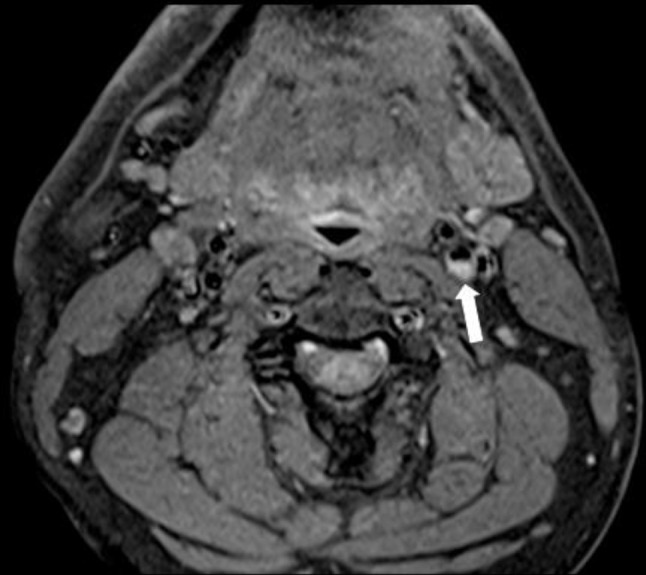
39-year-old male patient who presented with an acute left M1 occlusion with a background history of a prior left MCA infarct. Axial fat-saturated T1W MRI: crescentic hyper intense signal at the posterior aspect of the origin of the left ICA consistent with haemorrhage within the known carotid web. ICA, internal carotid artery; MCA, middle cerebral artery; T1W, *T*
